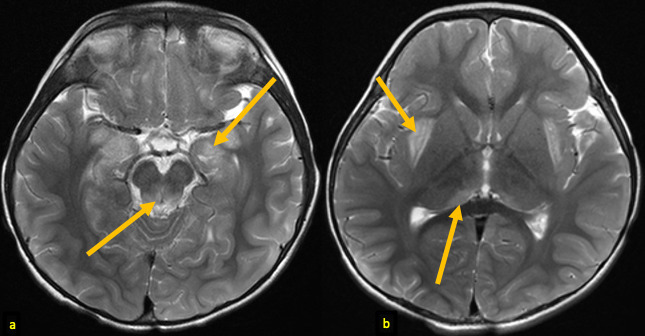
A 25-month-old male with AHNE. Initial brain MRI obtained during his first presentation for AHNE: axial *T*
